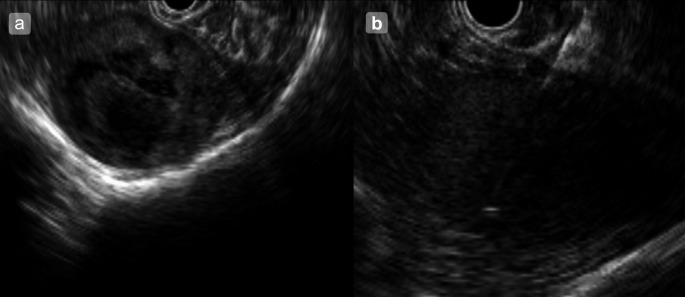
a EUS reveals a well-demarcated, hypoechoic splenic lesion with a heterogeneous internal echotexture. b EUS-TA was conducted using a 22-gauge Franseen-tip needle (Acquire; Boston Scientific, Marlborough, MA, USA); for the first pass, we used suction, but owing to initial blood contamination, subsequent passes were performed with the slow-pull technique; no immediate complications occurred. EUS: endoscopic ultrasound; EUS-TA: endoscopic ultrasound-guided tissue acquisition
